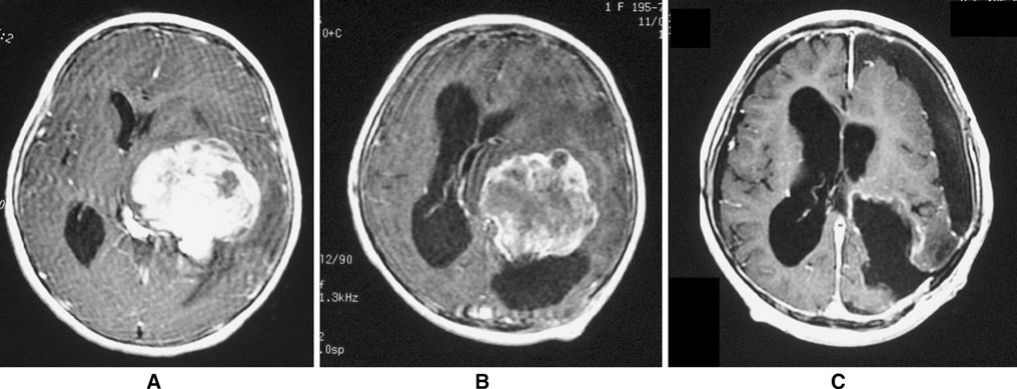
a Axial T_1_-weighted magnetic resonance images with gadolinium showing a large tumor in the left lateral ventricle. b Gadolinium-enhanced T1-weighted magnetic resonance image following irradiation to the tumor, showing noticeable diminution in enhancement. c Postoperative gadolinium-enhanced T_1_-weighted magnetic resonance image showing total tumor excision in the left lateral ventricle
